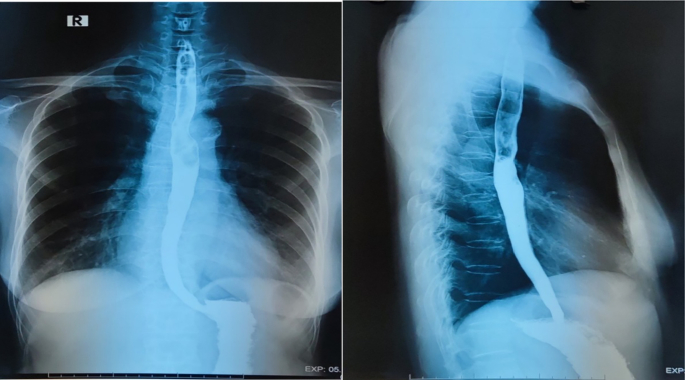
The barium swallowing X-ray at three months of follow up.
